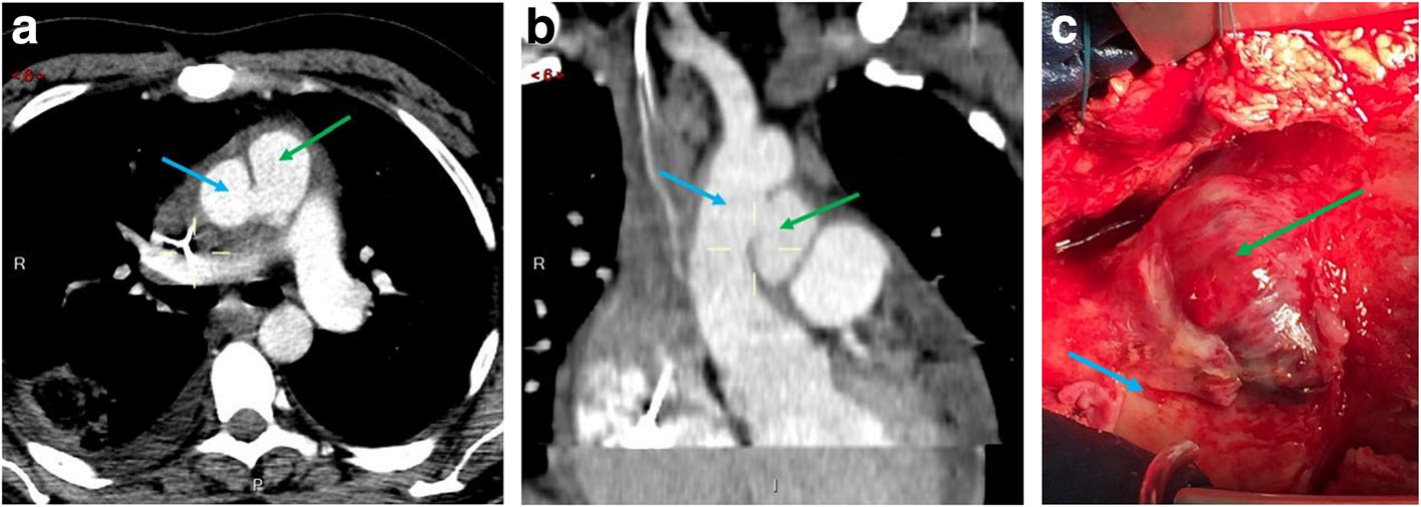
a and b computed tomography scan views of the false aneurysm (green arrow) and its relationship with the aorta (blue arrow). c per-operative view of the aneurysm (green arrow) and the aorta (blue arrow). Patient’s head is oriented towards left of the picture
